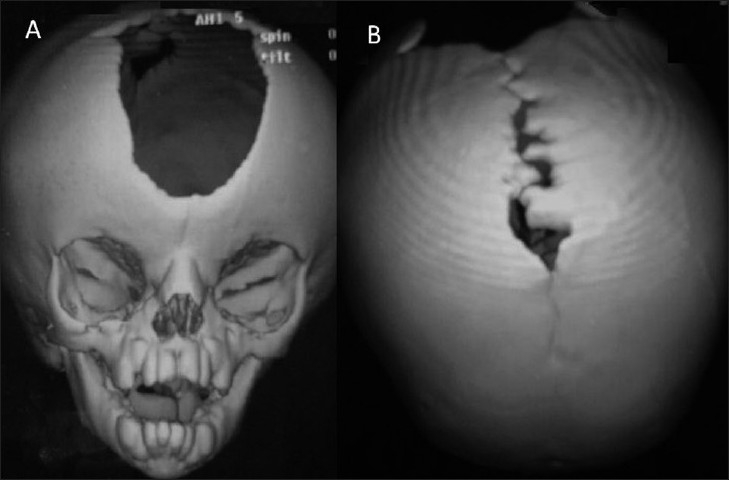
Case 1: (A and B) Three-dimensional reconstructed computed tomography scan showing a widely open anterior fontanelle with an open sagittal suture, bilateral coronal synostosis and a brachycephalic head
